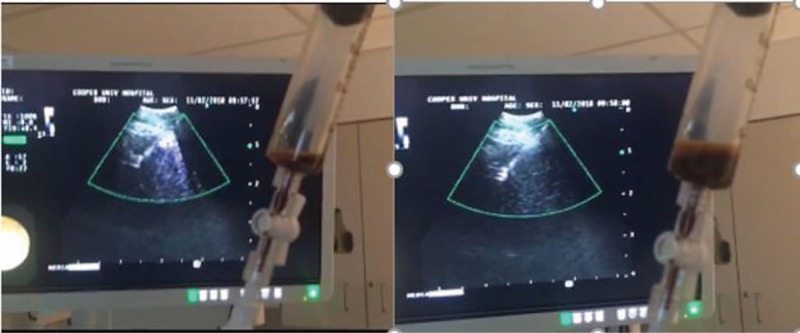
Transesophageal needle aspiration with EBUS scope. EBUS = endobronchial ultrasound.
